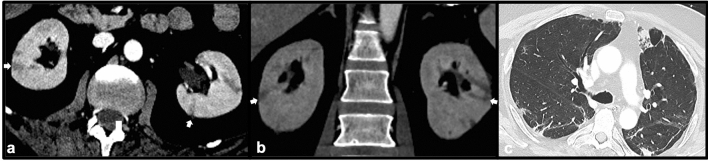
66-year-old male with COVID-19 infection and multiorgan failure requiring renal replacement therapy. Axial (a) and coronal (b) post-contrast CT images demonstrate multiple peripheral wedge-shaped cortical perfusion defects (white arrows) consistent with renal infarcts. Axial post-contrast CT in lung windows (c) demonstrates typical pulmonary findings of COVID-19 pneumonia
